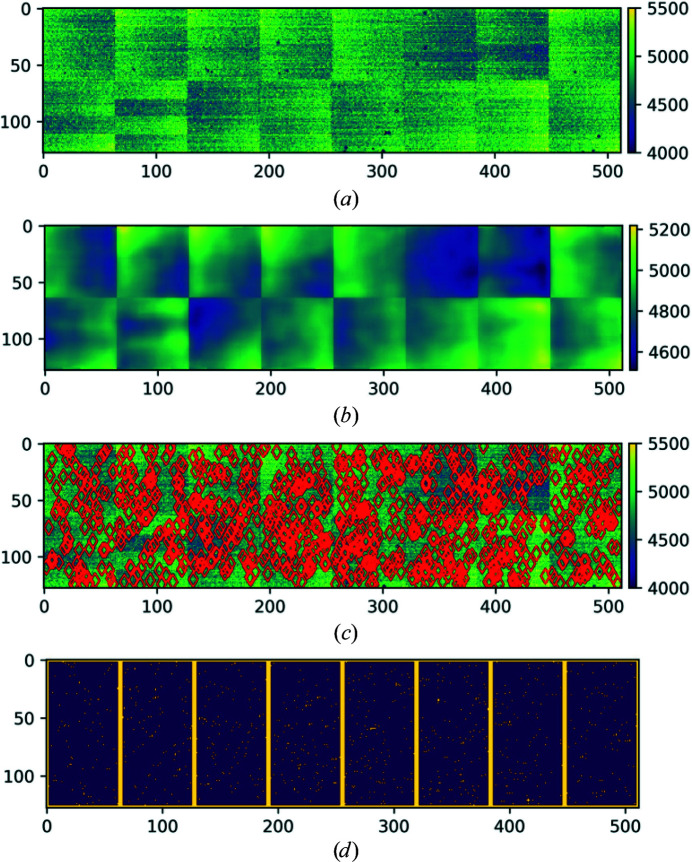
(*a*) The temporal average values of a module of an AGIPD-1M X-ray pixelated detector read in the absence of illumination. (*b*) The robust model values at the location of each pixel estimated to declare normal behaviour (estimated over pixels within 64 × 64 pixel windows). (*c*) The detected bad pixels are marked with red circles over the image from (*a*). (*d*) Detected bad pixels are shown in yellow. This figure has previously been published in Sadri *et al.* (2022 ▸).
